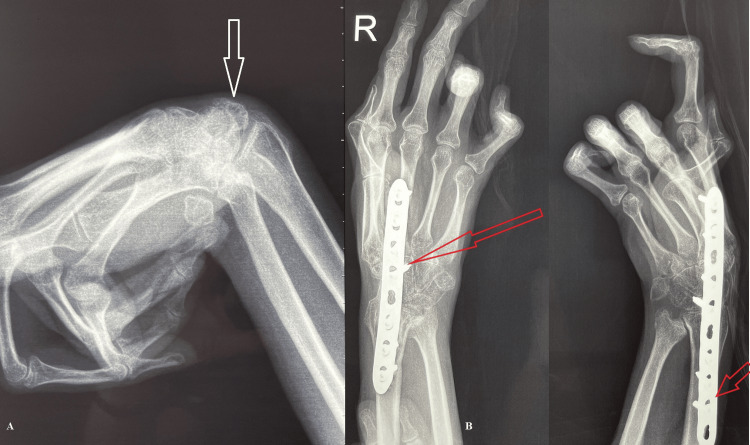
X-ray of the right hand A: Preoperative (white arrow: wrist contracture). B: Postoperative (red arrow: LCP). LCP: locking compression plate
